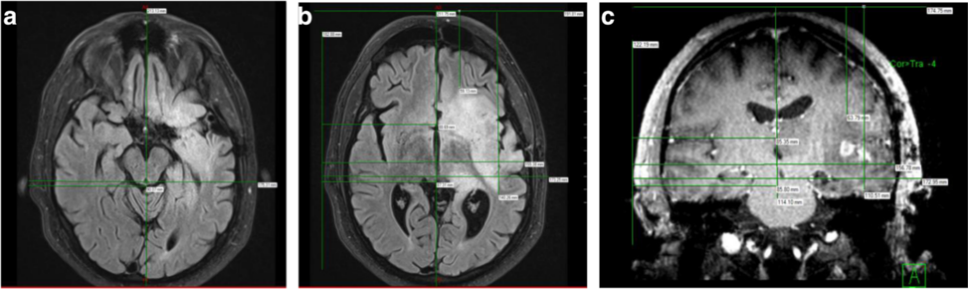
a, b & c show a largely noncontrast enhancing tumor. Head size measurements are performed on the FLAIR sequence. The axial coordinates for head size are shown in figure a. NovoTAL mapping was performed exclusively using figure b axial and figure c coronal FLAIR images. In the axial view figure b, a reference frame is drawn on the slice demonstrating the greatest extent of FLAIR abnormality. Tumor coordinates are obtained from the right frame to the near and far edges of FLAIR abnormality at the discretion of the treating physician. In figure c, coronal tumor location coordinates are obtained from a reference frame drawn at the level of the scalp which extends inferiorly to the lowest margin of temporal lobe. Distances to the tumor margin are drawn at the discretion of the treating physician to include all the region that is thought to contain active tumor
